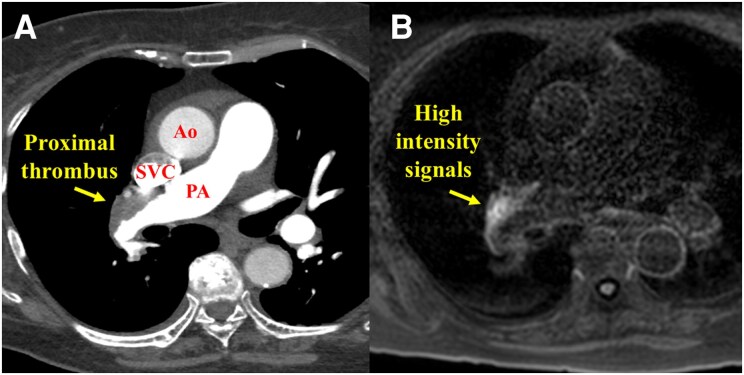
CTPA and MRDTI images at the patient’s initial referral. (*A*) Large mural thrombi were seen in the proximal right pulmonary artery on CTPA. (*B*) High-intensity signal on MRDTI at the area of the mural thrombi detected by CTPA, which suggested a possibility that this thrombus was not chronic. CTPA, computed tomography pulmonary angiography; MRDTI, magnetic resonance direct thrombus imaging; Ao, ascending aorta; SVC, superior vena cava; PA; pulmonary artery.
